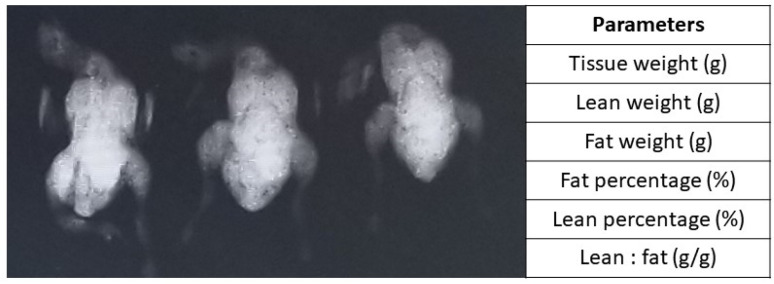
Scanned images of dual-energy X-ray absorptiometry (DEXA) of broiler chickens and corresponding parameters.
